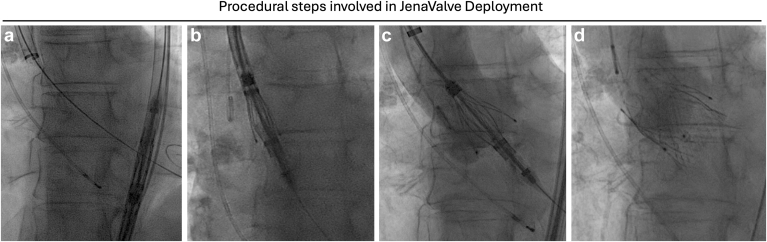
Procedural steps involved in JenaValve deployment. (a) The 22F 65 cm long sheath is advanced to the sinotubular junction. (b) The valve delivery system advanced through the sheath into the ascending aorta. (c) Locators are exposed and advanced to the AV cusps. The locator position is confirmed by aortic root angiography and transesophageal echocardiography. (d) Once positioning of locators and valve depth is confirmed, the valve is deployed. Abbreviation: AV, aortic valve.
